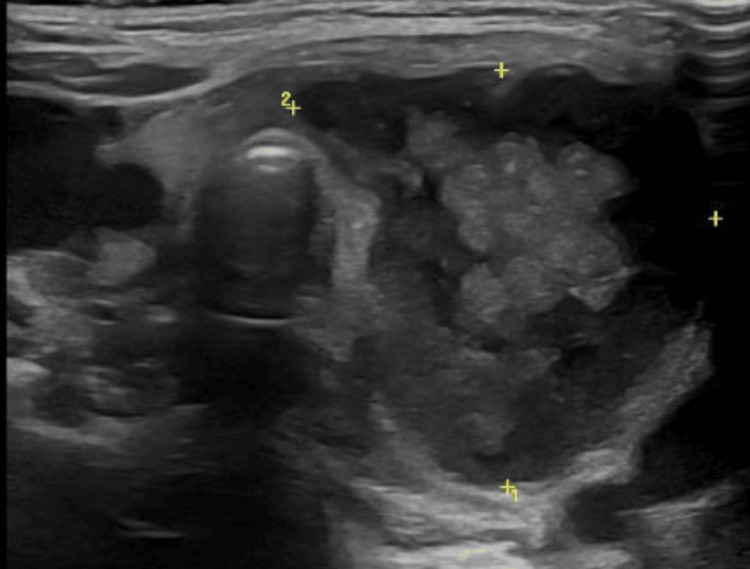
Thyroid ultrasound Thyroid ultrasound revealed a lobed heterogeneous iso- and hypoechoic mass, sized 2.8x3.0x3.7 cm, at the left thyroid lobe.
